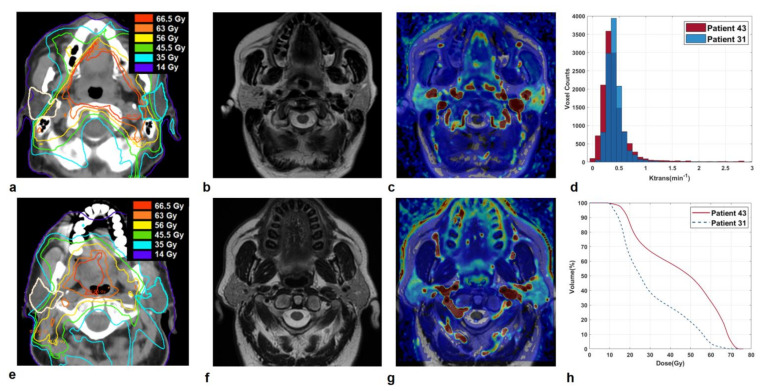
Sections of parotid glands in a 47-year-old woman affected by a base of the tongue carcinoma (Patient 43) on axial CT image with overlaid isodose levels and parotid contours (a); the corresponding axial T2-weighted image (b) and map of K^trans^ (c). Analogously, sections of parotid glands in a 69-year-old man affected by a base of the tongue carcinoma (Patient 31) on axial CT image with overlaid isodose levels and parotid contours (e); the corresponding axial T2-weighted image (f) and map of K^trans^ (g). K^trans^ histograms (d) and dose-volume histograms (h) of both parotids, in comparison.
